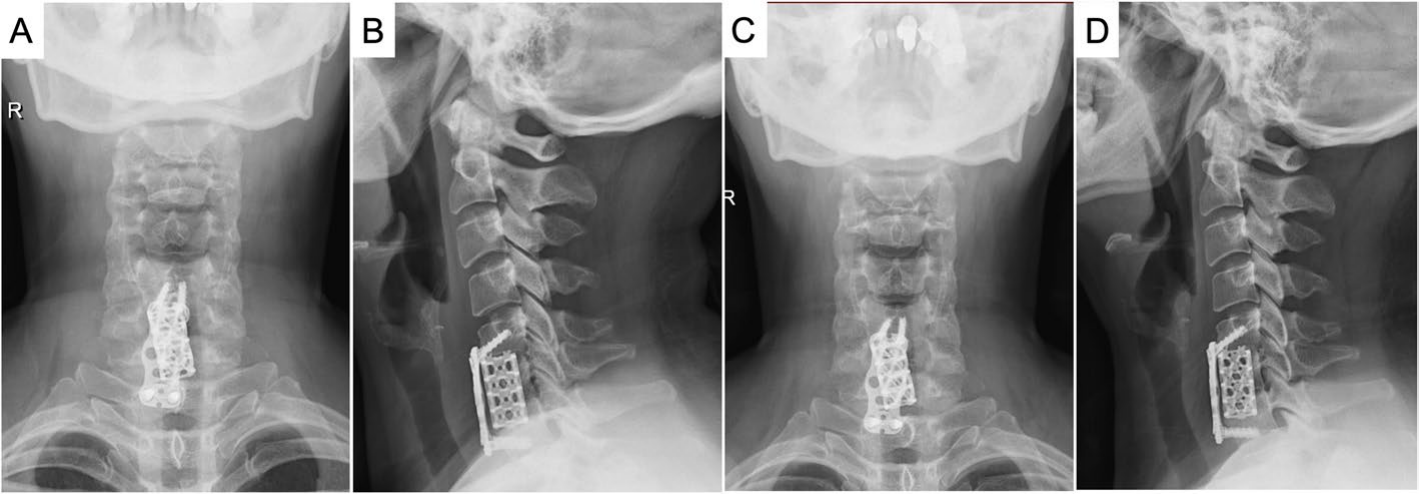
Cervical spine plain radiography taken at postoperative months 2 (A and B) and 6 (C and D). A and C anterior-posterior view; B and D lateral view
